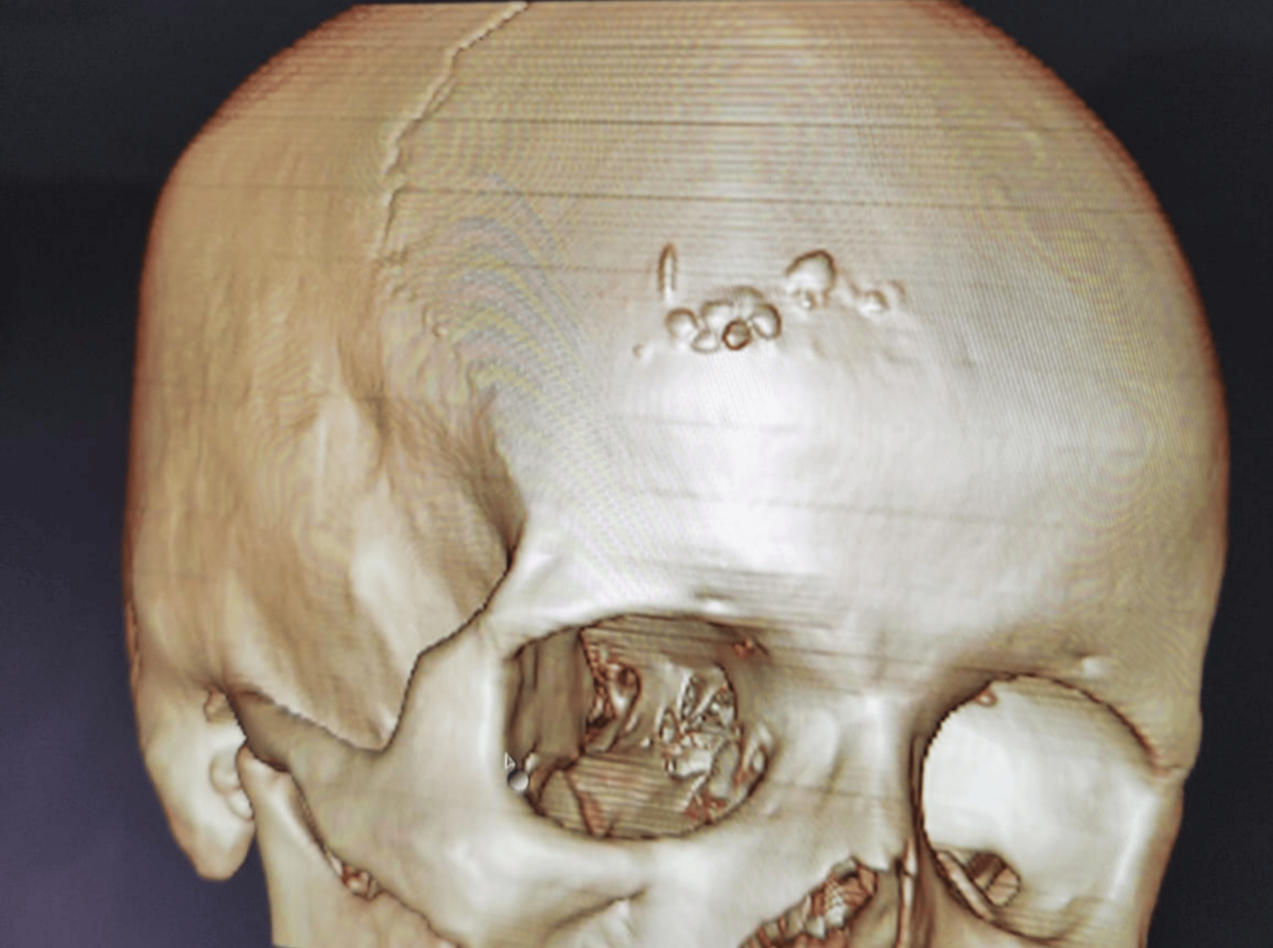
3D CT reconstruction image showing multiple irregularly hyperdense areas in the right frontal bone region CT: computed tomography
